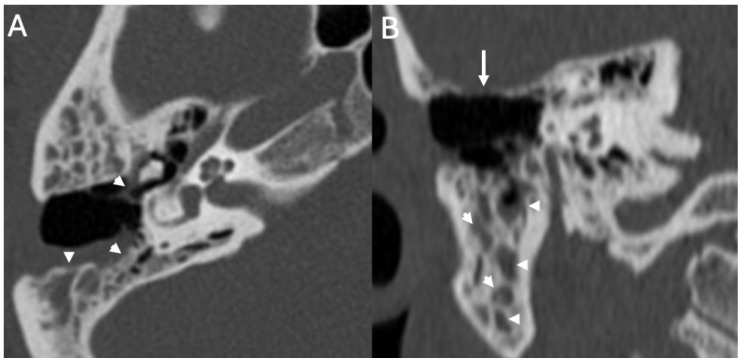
Menigitis due to *Streptococcus pneumoniae* in a 33-year-old. Axial (A) and coronal CT (B) showing right otomastoid (arrowheads in (A,B)) and erosion of the tegmen tympani (arrow in (B)) The patient also underwent an open tympanoplasty due to cholesteatoma 10 years beforehand.
